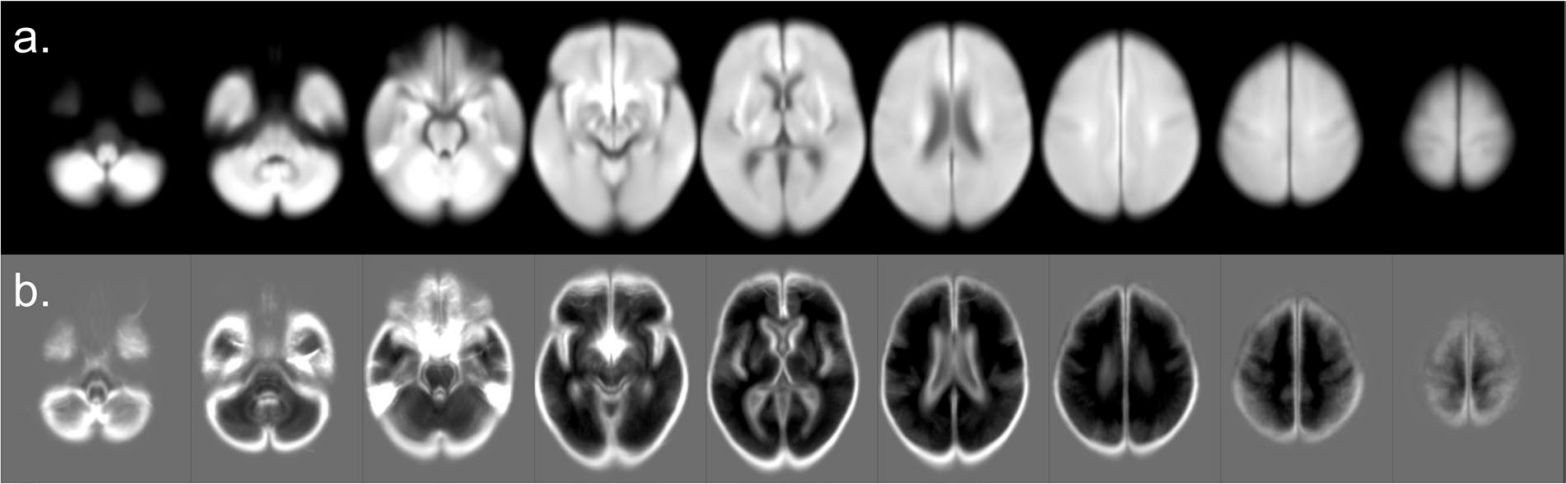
Radiological normal DWI templates. (a) average and (b) standard deviation maps of the intensity-normalized DWIs in standard space (MNI) of the cases classified as “not-visible” strokes.
